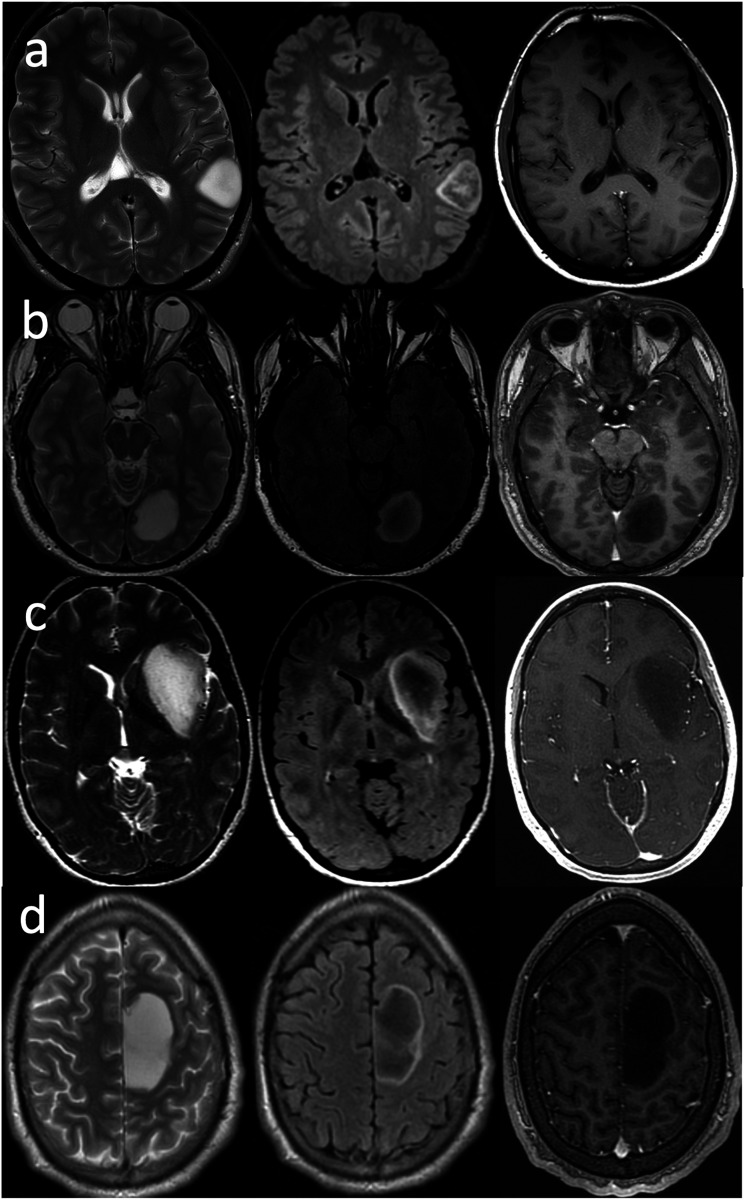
IDH-mutant, 1p/19q non-codeleted diffuse gliomas in two 37-year-old males (a), (b), a 31-year-old female (c), and a 31-year-old male (d). All demonstrate the strict criteria of T2FMM: complete or near-complete homogeneous hyperintensity on T2WI (left column), relatively hypointense signal on FLAIR, except for a thin hyperintense peripheral rim (middle column), and little-to-no enhancement on postcontrast T1WI (right column). Of note, tumor “a” exhibits an intrinsic central hyperintensity on the pre-contrast T1WI (not shown) and does not enhance.
